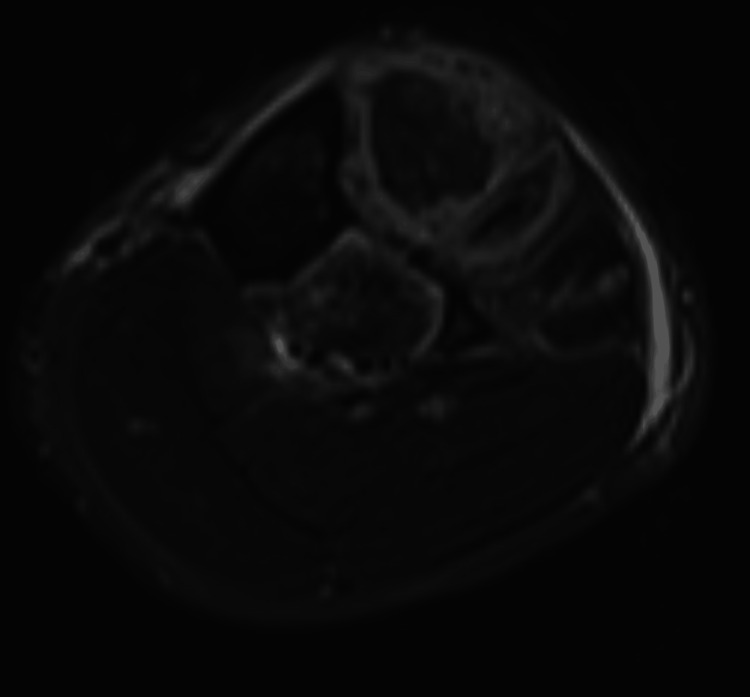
Axial MRI of left leg day eight post-index procedure. Compartmental oedema of the anterior and deep posterior compartments
